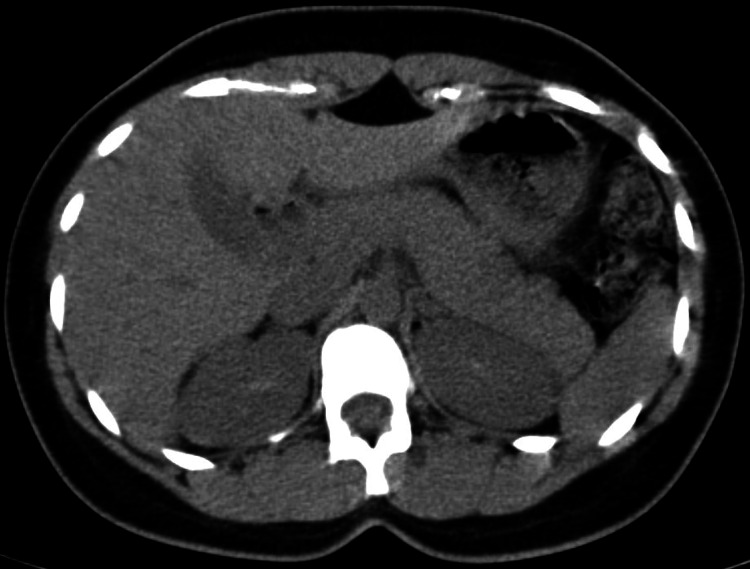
CT abdomen of patient 4, showing a normal pancreas
